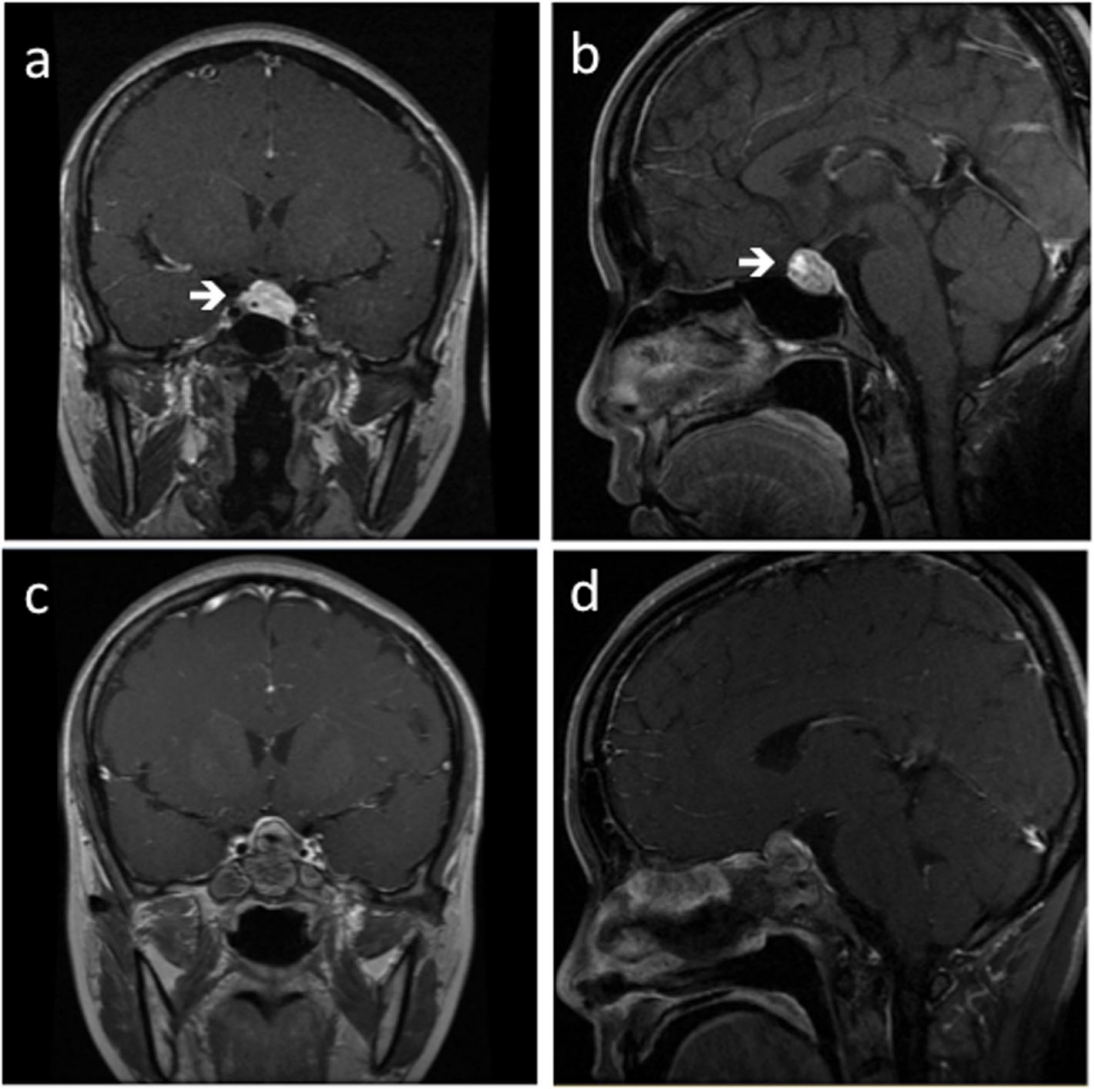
a Coronal view of cranial contrast-enhanced MRI before operation (white arrow). b Sagittal view of cranial contrast-enhanced MRI before operation (white arrow). c Coronal view of cranial contrast-enhanced MRI of this patient in 3 months after operation. d Sagittal view of cranial contrast-enhanced MRI of this patient in 3 months after operation
